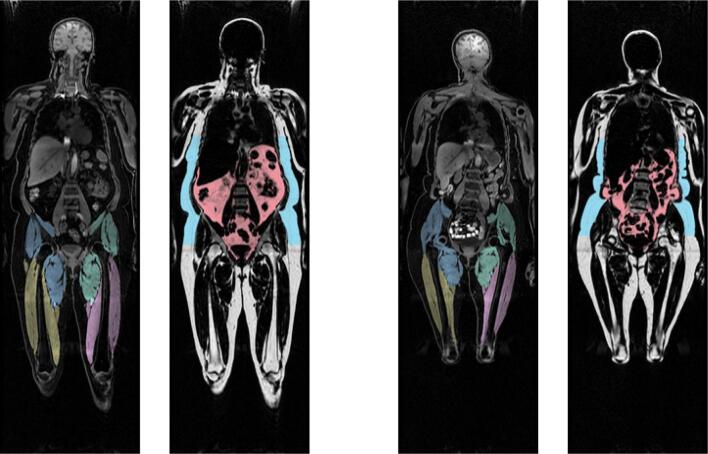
Body MRI. Showing two participants with a coronal slice from their MRI scan with VAT (pink) and ASAT (blue), and thigh muscle segmentations. (For interpretation of the references to colour in this figure legend, the reader is referred to the web version of this article.)
